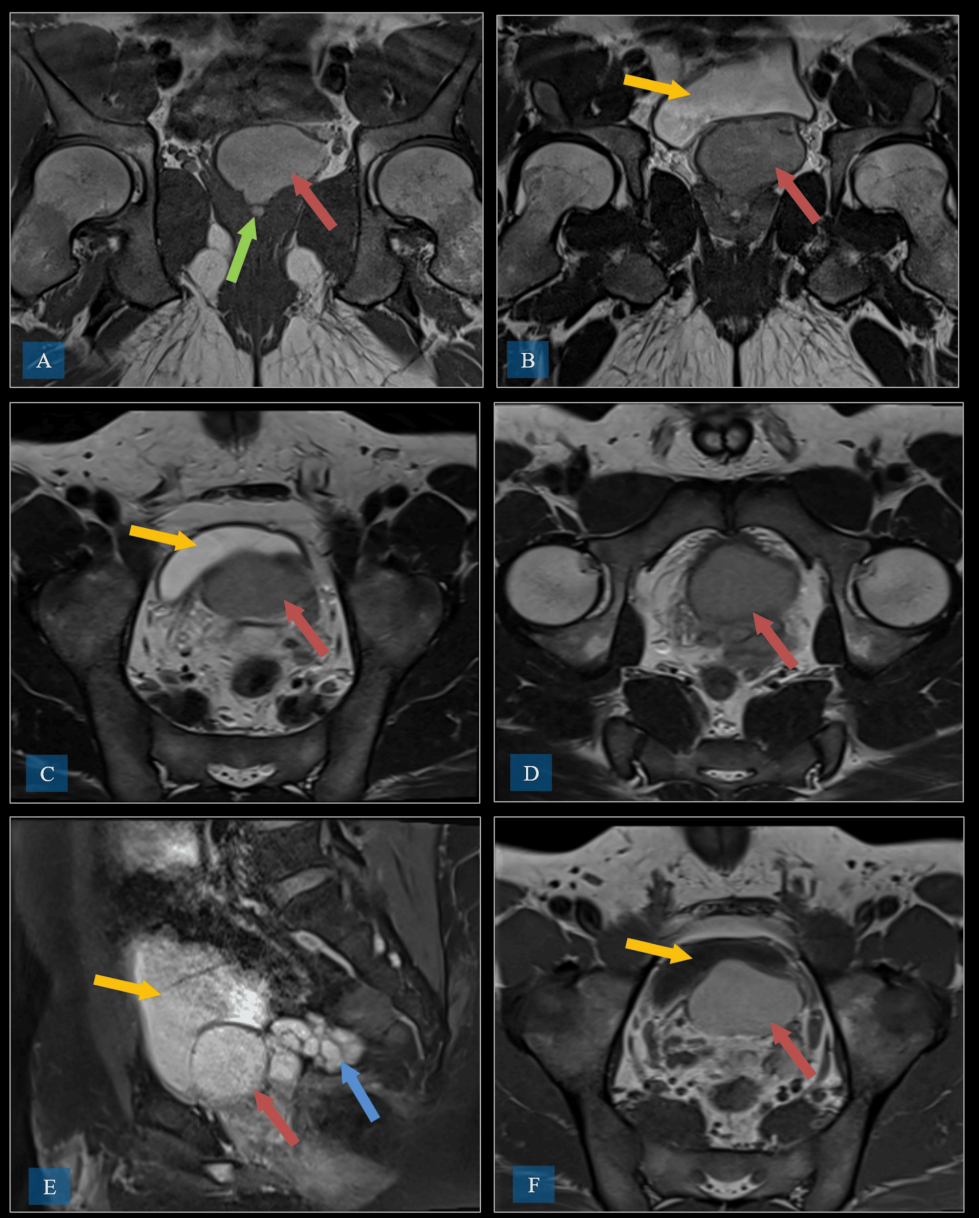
MRI of the pelvis demonstrating a large convoluted cystic dilatation of the left seminal vesicle measuring approximately 6 cm in diameter. (A) Coronal T1-weighted MRI. The red arrow indicates the left seminal vesicle cyst, and the green arrow indicates the ejaculatory duct obstruction. (B) Coronal T1-weighted MRI. The red arrow indicates the left seminal vesicle cyst, and the yellow arrow indicates the urinary bladder. (C) Axial T2 TSE. The red arrow indicates the left seminal vesicle cyst, and the yellow arrow indicates the urinary bladder. (D) Axial T2 TSE. The red arrow indicates the left seminal vesicle cyst. (E) Sagittal T1 TSE. The red arrow indicates the left seminal vesicle cyst, the yellow arrow indicates the urinary bladder, and the blue arrow indicates the dilated left seminal vesicle. (F) Axial T1 TSE. The red arrow indicates the left seminal vesicle cyst, and the yellow arrow indicates the urinary bladder. TSE: turbo spin echo
